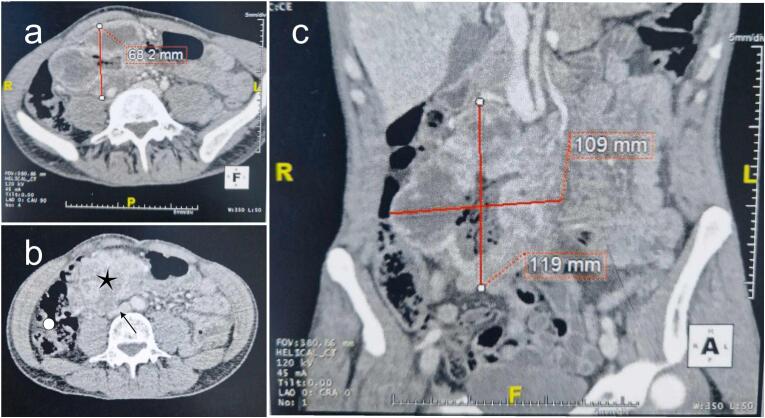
Preoperative computed tomography findings. (a), (b) axial views and (c) coronal view, showing a small bowel tumor (Black star in (b)), encompassing a necrotic region, and exerting pressure on the inferior vena cava (Black arrow in (b)) and the adjacent ascending colon (White circle in (b)).
